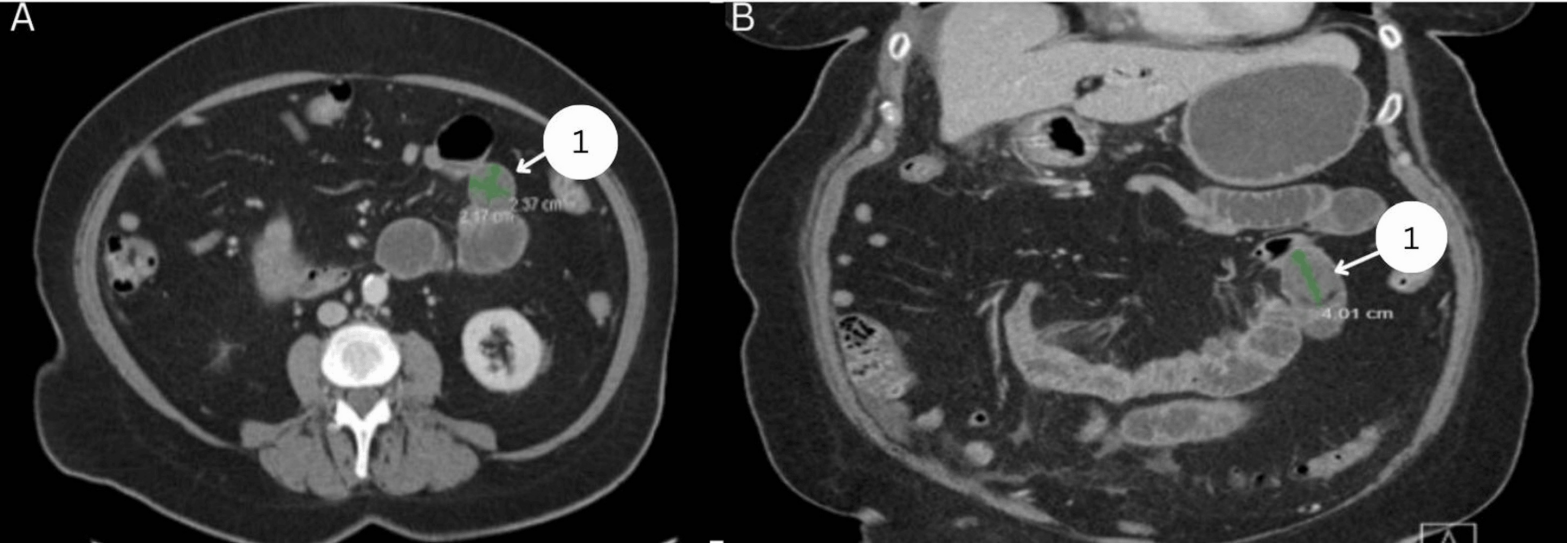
Preoperative CT images for patient 2 in the axial and coronal views 1: 4 cm gallstone in the loop of the jejunum CT: computed tomography
